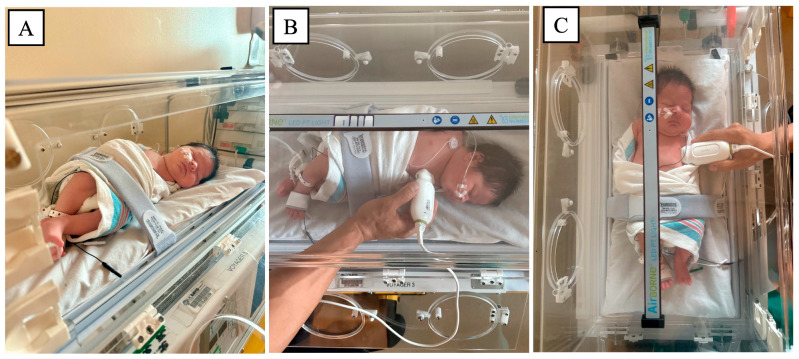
Proposed positioning and probe placement techniques for neonatal point-of-care ultrasound (POCUS) performed within an incubator. (A) Left side-lying positioning with low swaddle and low belt to optimize POCUS acquisition. (B) Cardiac probe placement in the left parasternal position within the incubator. (C) Lung ultrasound probe placement for anterior lung views while maintaining incubator constraints.
